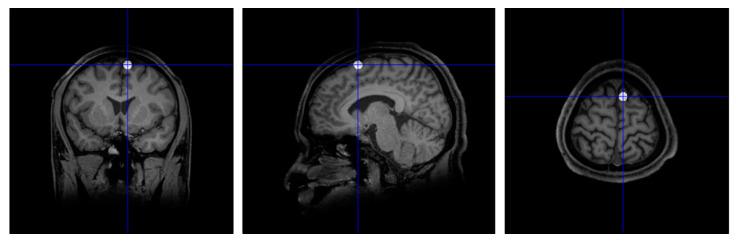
The position of right pre-supplementary motor area (pre-SMA) marked on the individual MRI scan.
